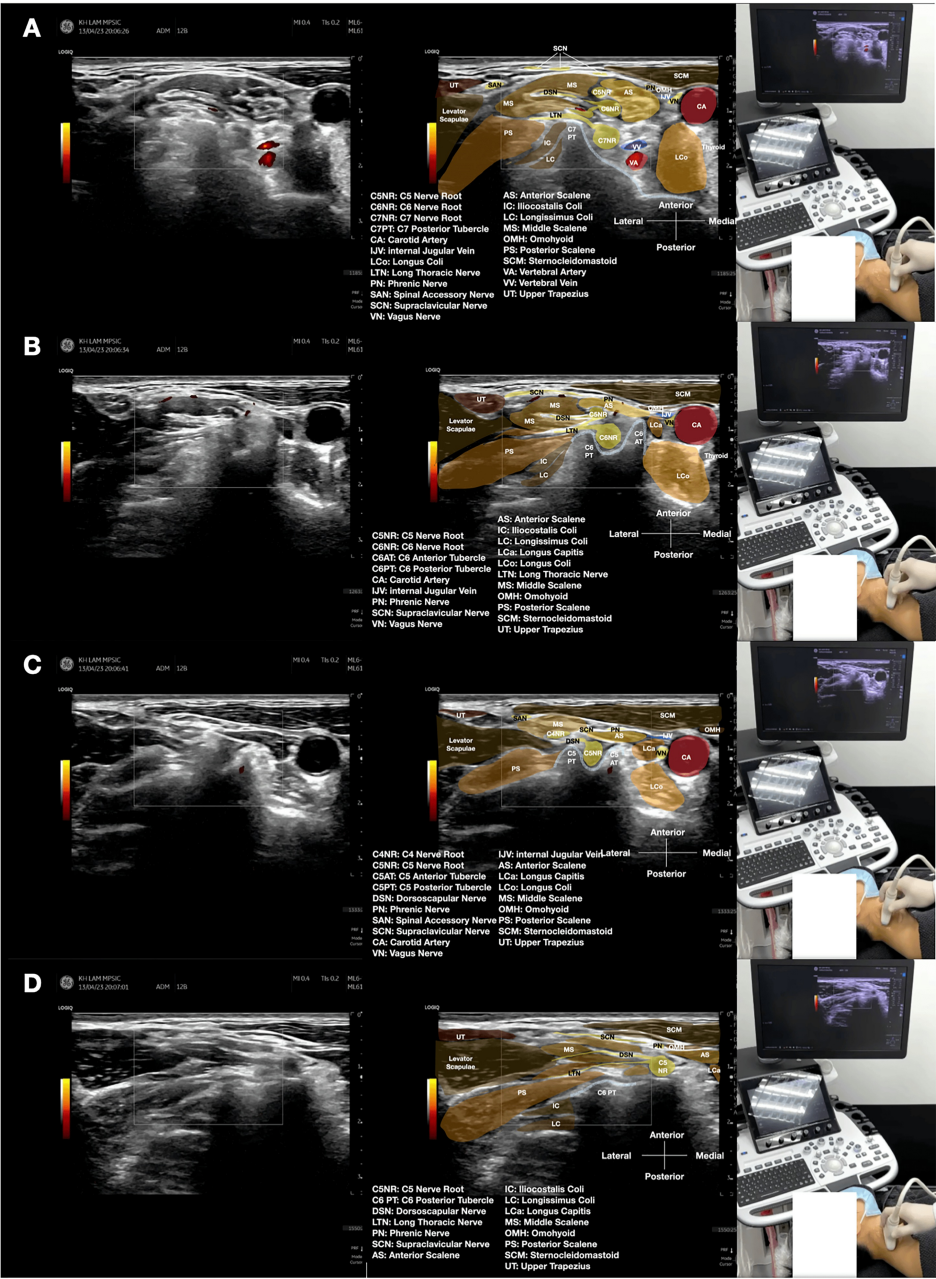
Sonoanatomy of the dorsal scapular nerve in the middle scalene muscle. Ultrasound images demonstrating the anatomical relationships for dorsal scapular nerve (DSN) identification at the scalene level. (A) C7 nerve root level showing characteristic anatomy with a prominent posterior tubercle. (B) C6 nerve root level emerging between prominent anterior and posterior tubercles. (C) C5 nerve root level, the origin of the DSN. (D) The DSN is visualized within the middle scalene muscle as a hypoechoic oval structure (short axis) or fascicular structure (long axis). Key anatomical features include: the middle scalene muscle appearing as a hypoechoic, striated structure; cervical nerve roots as hypoechoic, round structures emerging from intervertebral foramina; and the DSN coursing through the middle scalene muscle (see Video 1).
